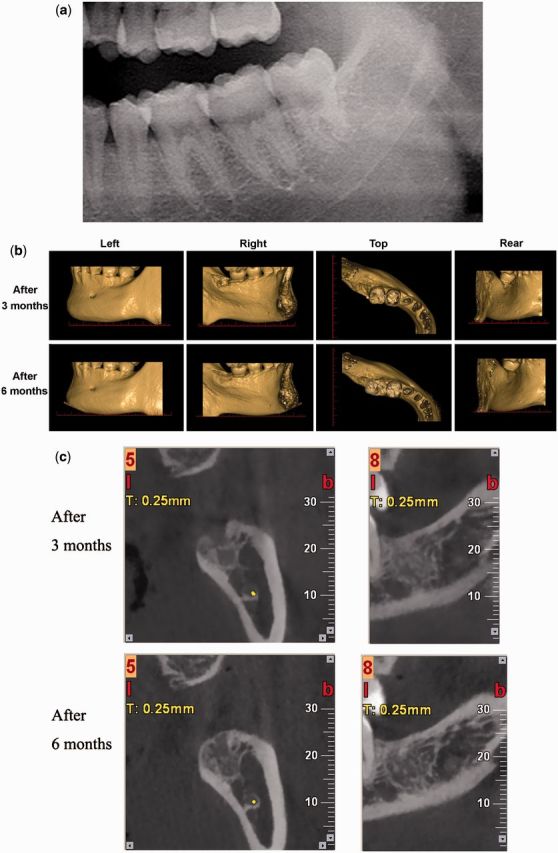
Radiographs of control site. (a) X-ray image before extraction; (b) CBCT 3D reconstructions at 3 and 6 months after extraction; (c) CBCT sections 3 months and 6 months after extraction.
